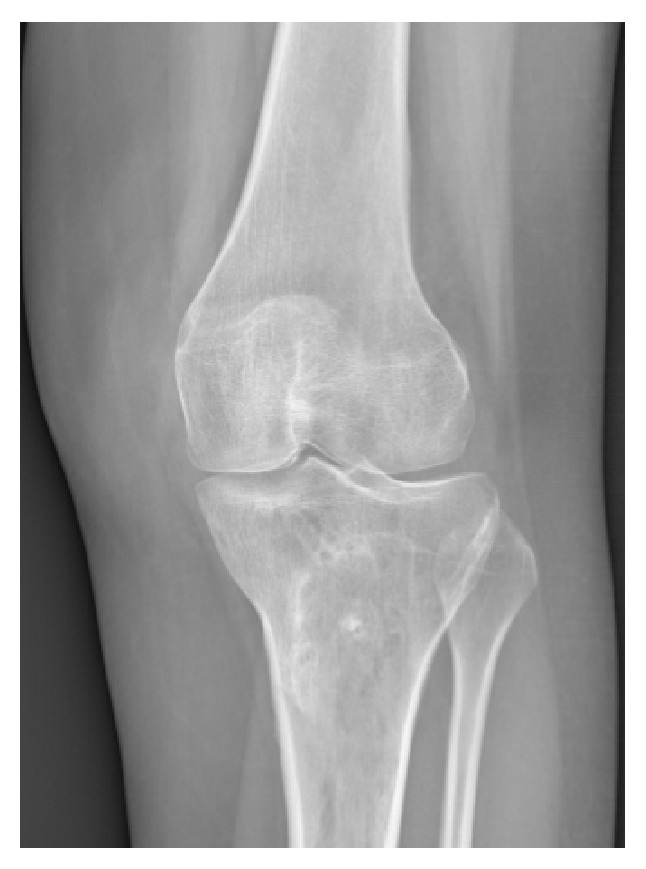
Post-op anteroposterior knee X-ray.
